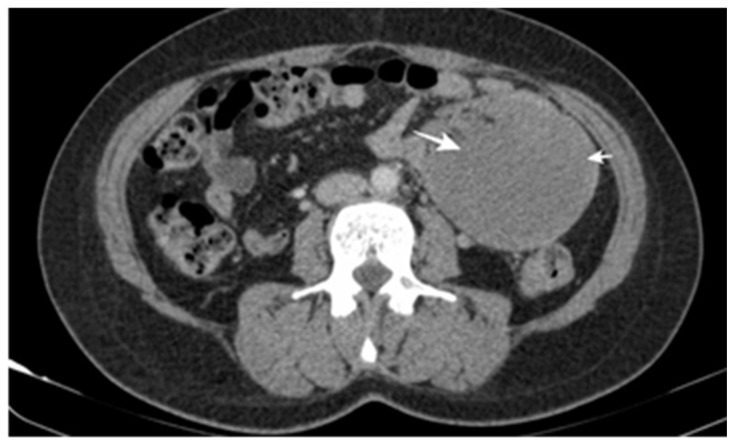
CT post-contrast axial scan: heterogeneous mass at the level of jejunal loops, round-shaped, with regular contours. The pathology exam revealed a mesenchymal angiomatous tumor.
